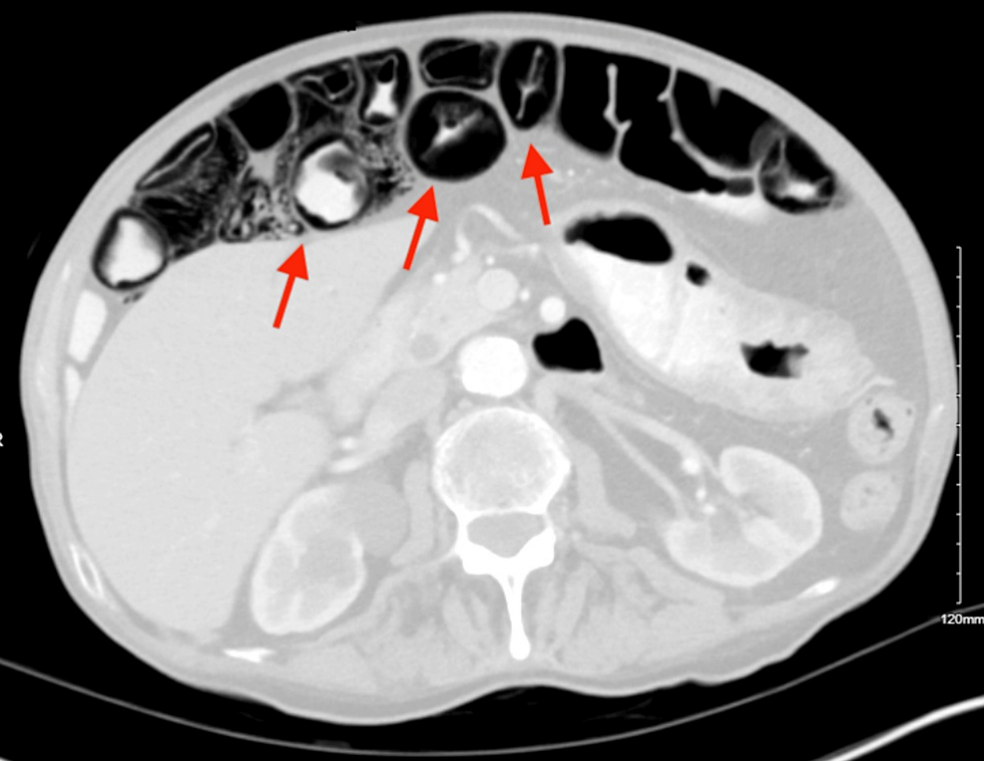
Prominent pneumatosis intestinalis depicted by arrows on the axial view of abdomen and pelvis computed tomography (CT).
